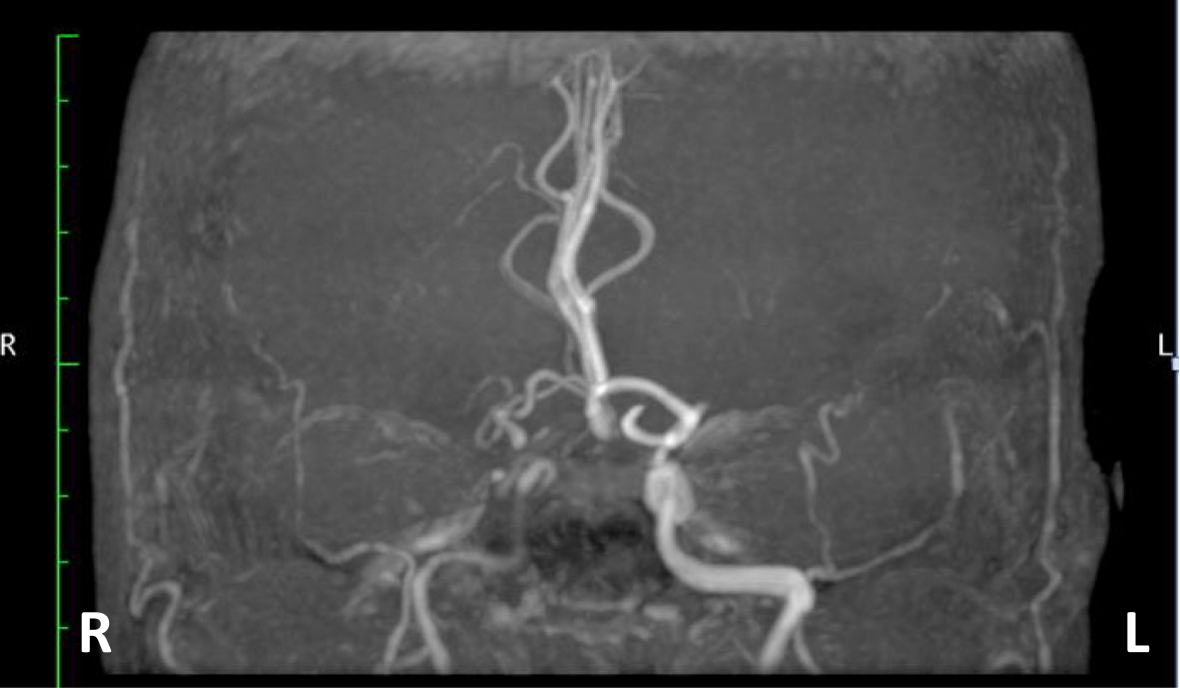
Magnetic resonance imaging (MRI) angiography. MRI demonstrated stenosis in bilateral internal carotid arteries, with occluded and poorly visible bilateral middle cerebral arteries
